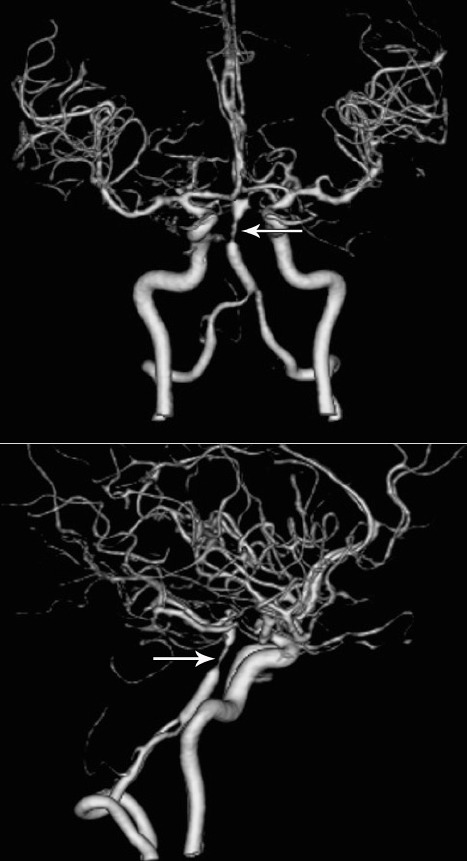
Anterior-posterior and lateral views CTA 3D reconstruction shows severe upper mid basilar artery vasospasm.
